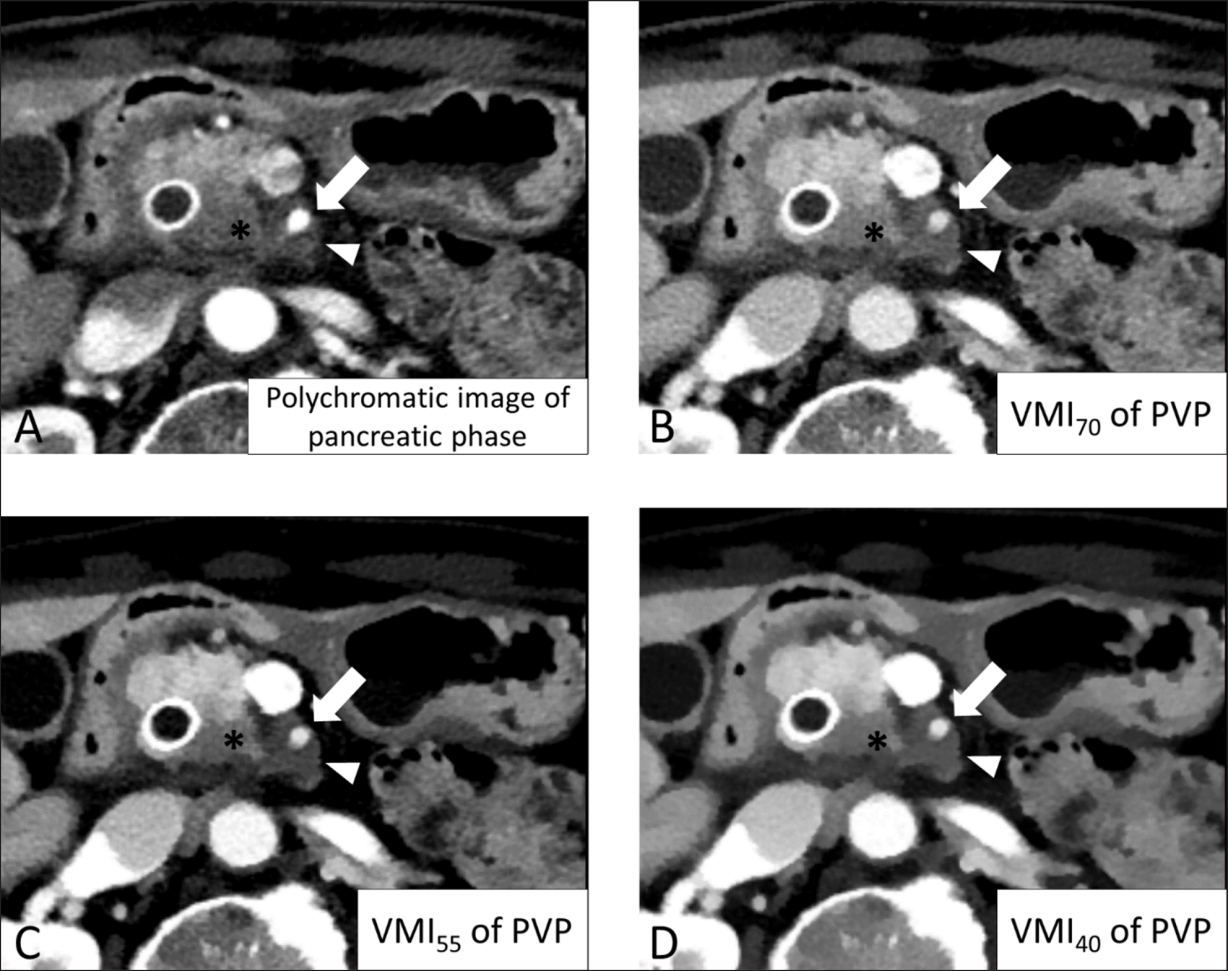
CT images of a 68-year-old man with pancreatic ductal adenocarcinoma (PDAC). The superior mesenteric artery (arrow) is well visualized on both VMI_40_ (B) and VMI_55_ of PVP (C), as well as on the pancreatic phase (A). PDAC (asterisk) and adjacent fat are more clearly demarcated on VMI_40_ (B) and VMI_55_ (C) of the PVP than on the pancreatic phase (A). The extent of tumor contact with the superior mesenteric artery (arrowhead) is more clearly visualized on VMI_40_ (B) and VMI_55_ (C) of the PVP than on the pancreatic phase. PVP: portal venous phase; VMI_40, 55, 70_: virtual monoenergetic image at 40, 55, and 70 keV of the PVP.
